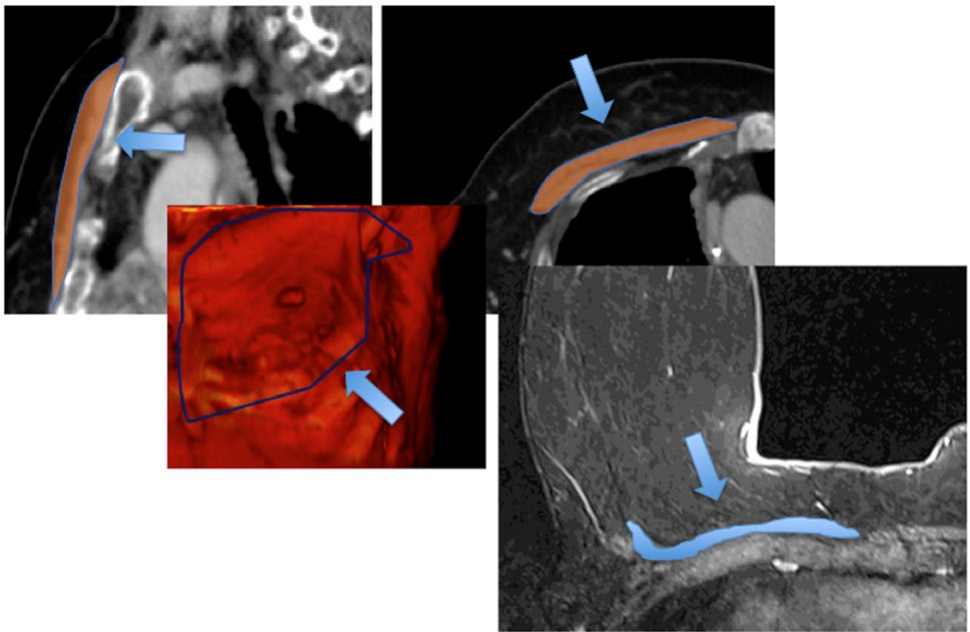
Thoracic CT and MRI images taken at the Lewis angle to estimate muscle mass on pectoralis muscle (arrows). Examples from patent number: 102019000016049
